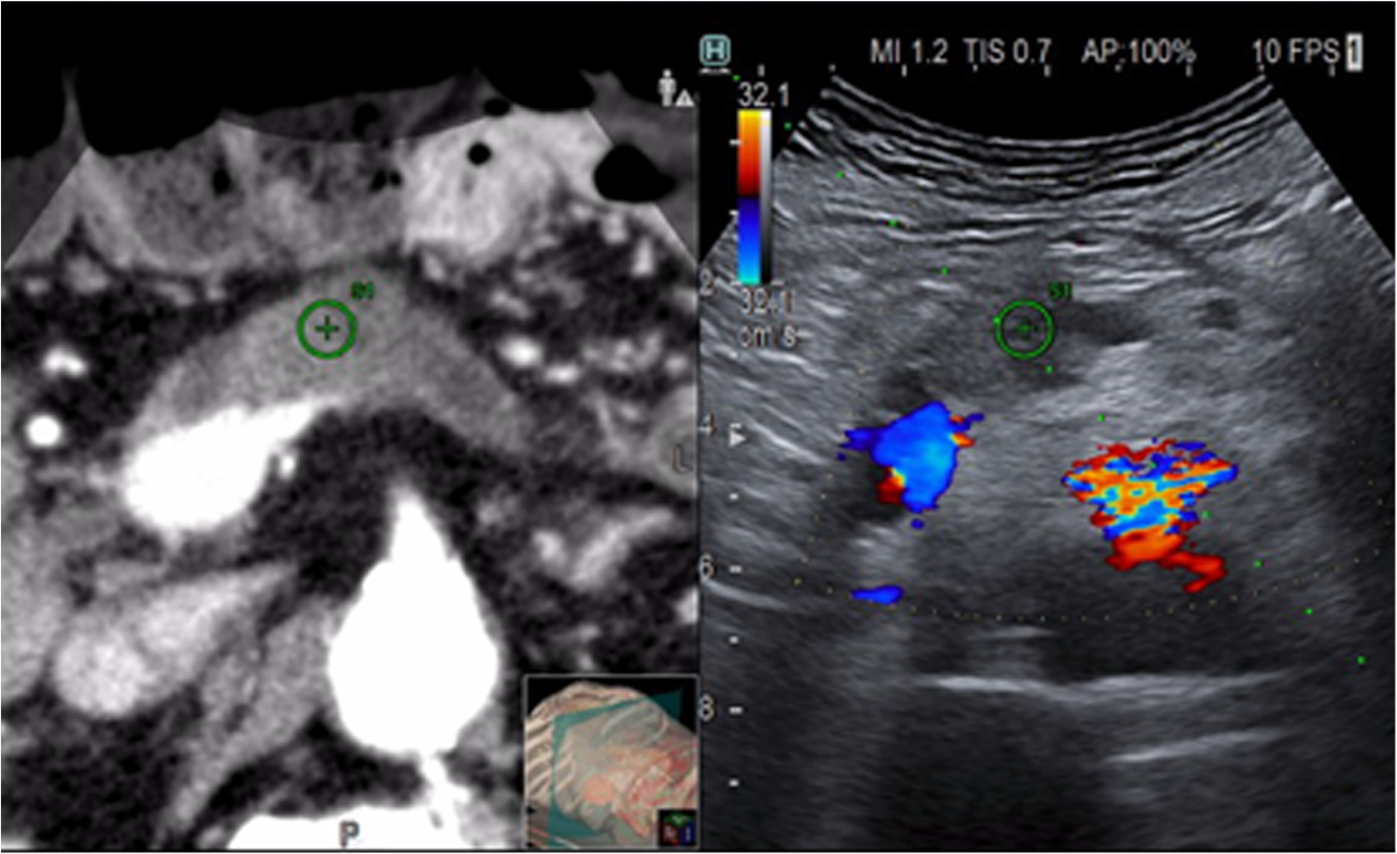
Ultrasound (US) image fused with a previously acquired computed tomography (CT). Target lesion easily is identified and marked (⊕) on both sides. Color Doppler confirms the major vessels’ relationship of the lesion well visualised on the CT on the left. Path of the needle precisely planned (*dotted line*). Interposed colon on the CT image is displaced on US by the strong compression applied by the probe
